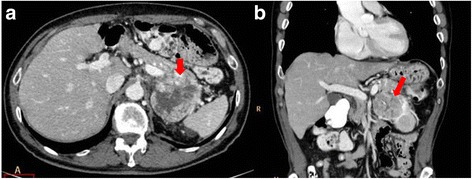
CT scan in axial plane (a) and coronal plane, MPR (b), during portal phase of dynamic contrast study. Tail pancreatic adenocarcinoma that infiltrates vascular hilum of the spleen
